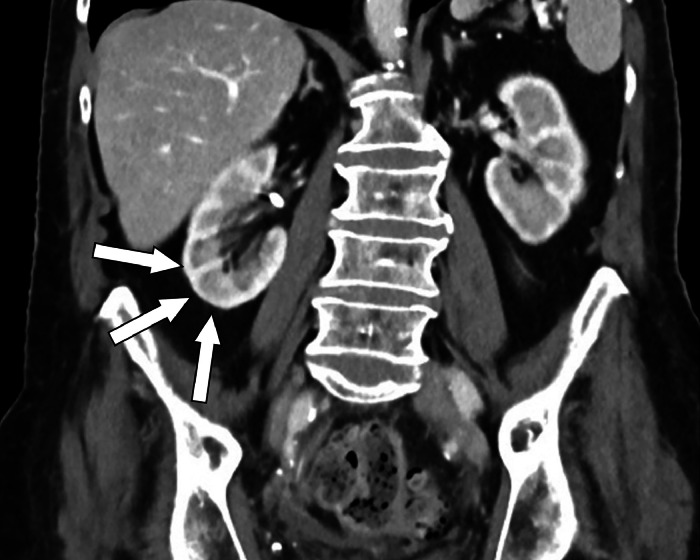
Follow-up imaging with contrast-enhanced abdominal CT on September 13th 2023. Contrast-enhanced abdominal CT in venous phase, coronal reformation. This CT showed no sign of renal mass on the right kidney (white arrows).
